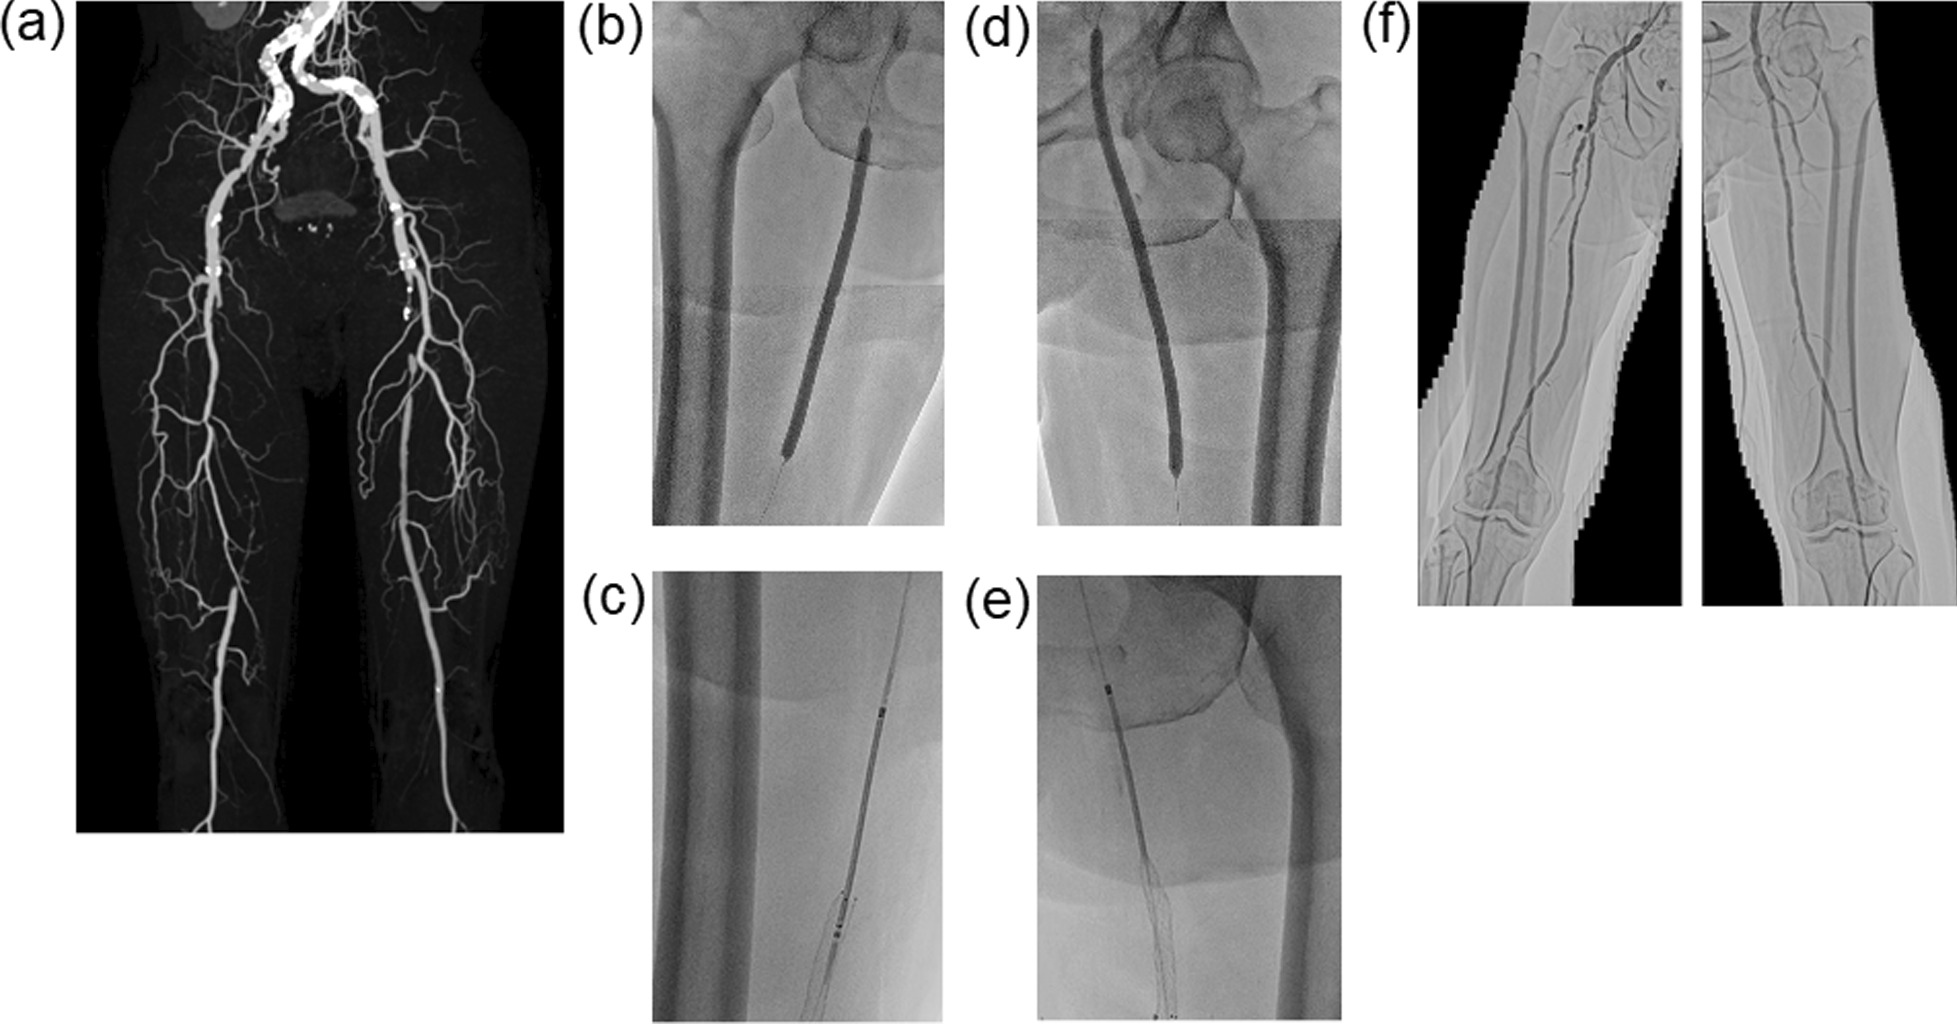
a Computed tomography (CT) angiographic image showing total occlusion of the right and left superficial femoral artery (SFA): from the ostium to the distal portion in the right SFA; from the ostium to the mid portion in the left SFA. Angiography of the right and left SFA before and after peripheral vascular intervention (PVI), shown in b–f. PVI in the right SFA was performed with balloon inflation b and stent implantation (c), and that in the left SFA was performed with balloon inflation d and stent implantation (e). Final angiography after PVI showing good results in both legs (f)
